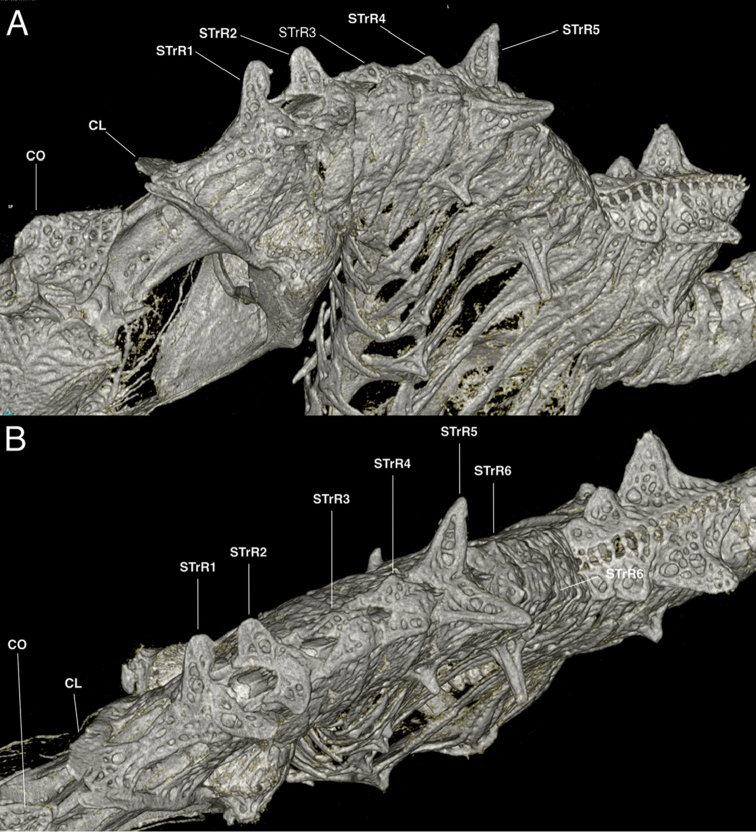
Computed tomography scanned anterior trunk area of *Hippocampuspontohi*, AMS I.47833-001, preserved male non-type, 16.33 mm SL, Cape Kri, Raja Ampat, Indonesia (photograph Graham Short). A Anterolateral view B Dorsal view. Note the double pair of spines projecting dorsolaterad on STrR1 and STrR1, respectively, and laterodorsal surface flat on STrR3, and STrR4. Abbreviations: CO, coronet; CL, cliethral ring; STrR1, first superior trunk ridge; STrR2, second superior trunk ridge; STrR3, third superior trunk ridge; STrR4, fourth superior trunk ridge; STrR5, fifth superior trunk ridge; STrR6, sixth superior trunk ridge.
